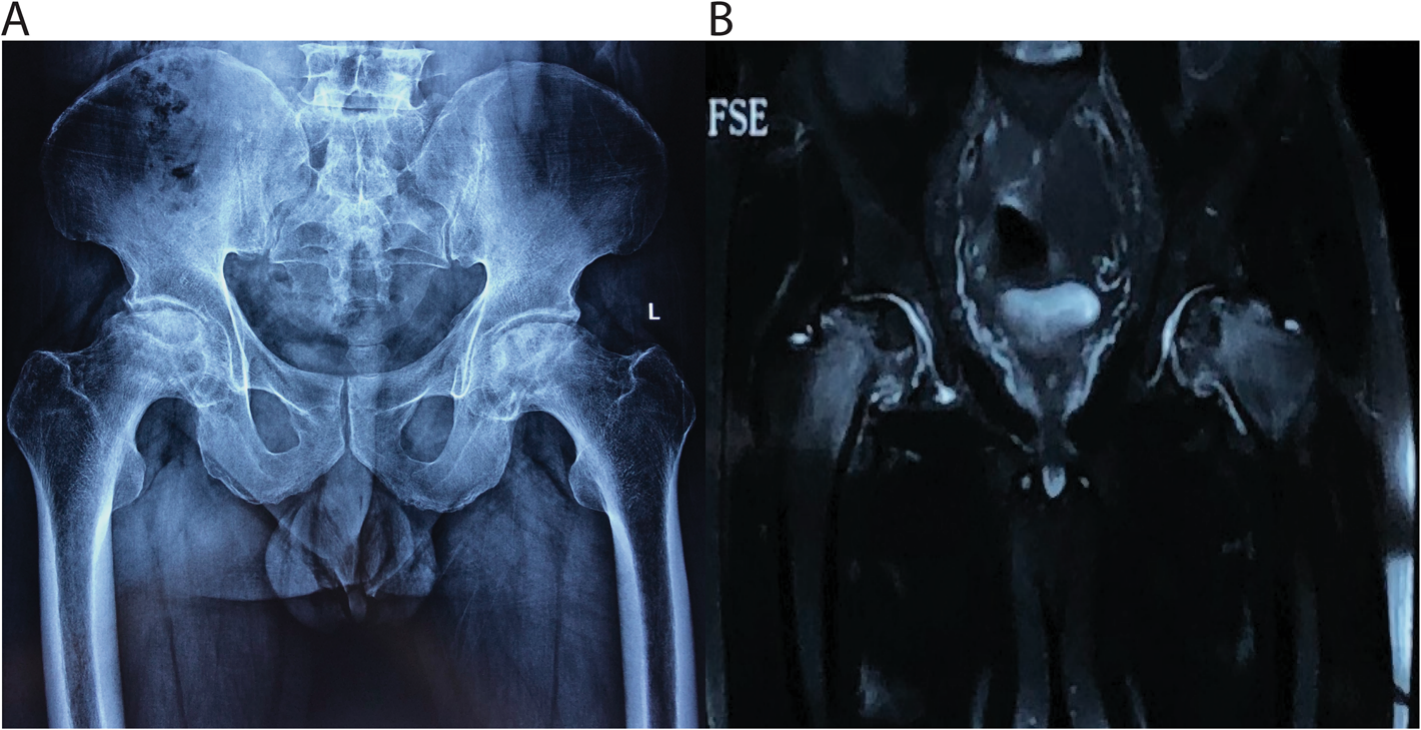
Both plain radiograph (a) and MRI (b) showed osteonecrosis on the bilateral side of the femoral head 1 year after the first diagnosis
